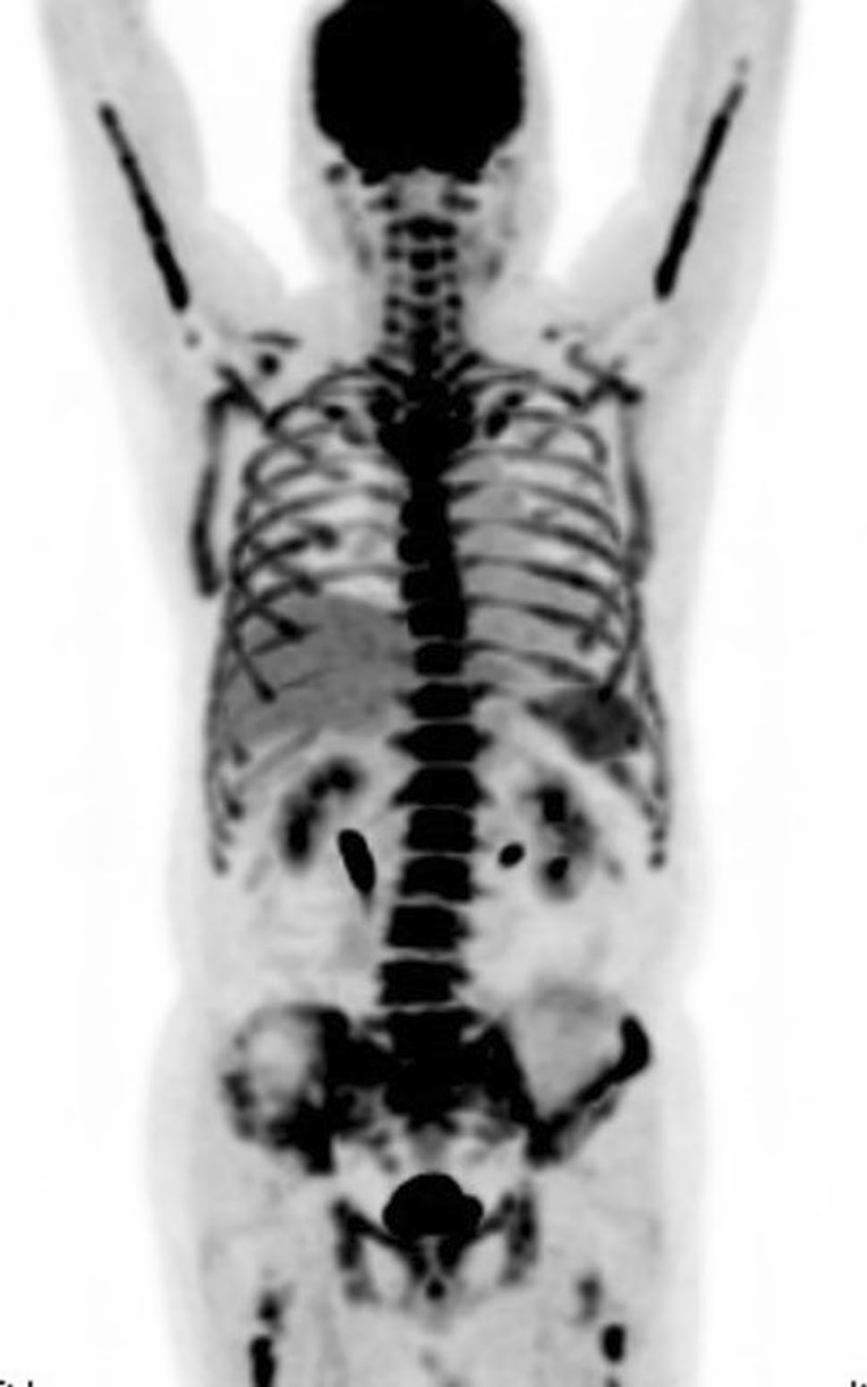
Marked increased uptake in the hematopoietic bone marrow and spleen in a patient with lymphoma, who received pegfilgrastim 7 days before the FDG–PET examination
